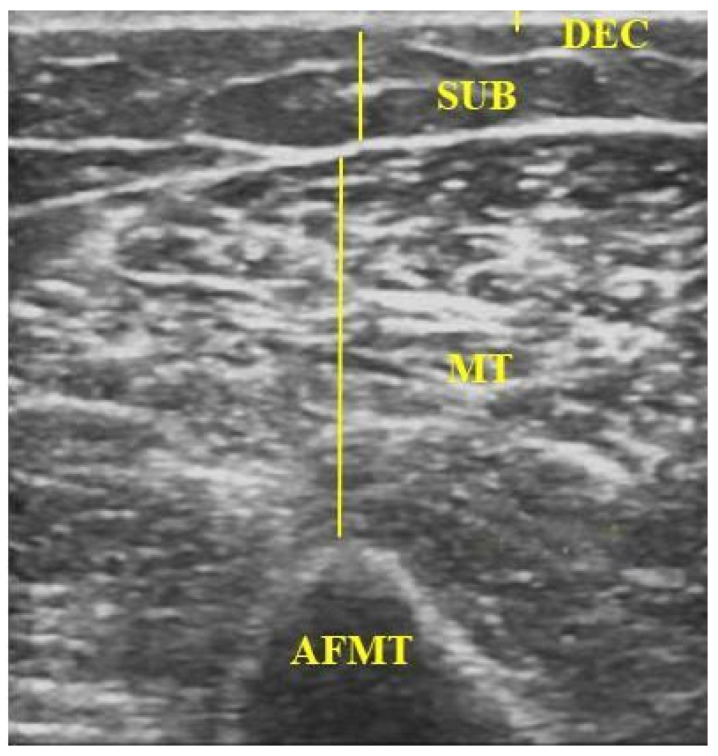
Ultrasound image showing measurements of DEC, SUB, and MT of the arm flexor muscles. DEC: dermal–epidermal complex; SUB: subcutaneous tissue; MT: muscle thickness; AFMT: arm flexor muscle thickness. Source: the authors (2025).
